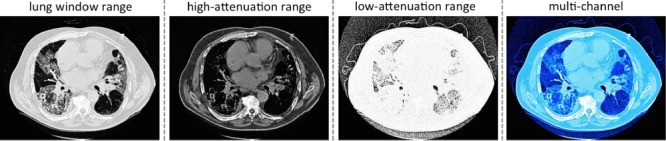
An example of lung/high-attenuation/low-attenuation CT windowing for an axis lung CT slice. We encode the lung/high-attenuation/low-attenuation CT windowing into red/green/blue channels.
